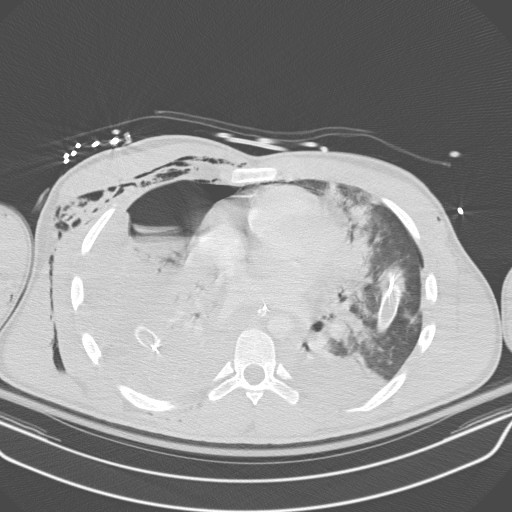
Contrast CT chest showed pneumopericardium, pneumomediastinum, right hydropneumothorax and subcutaneous emphysema.
